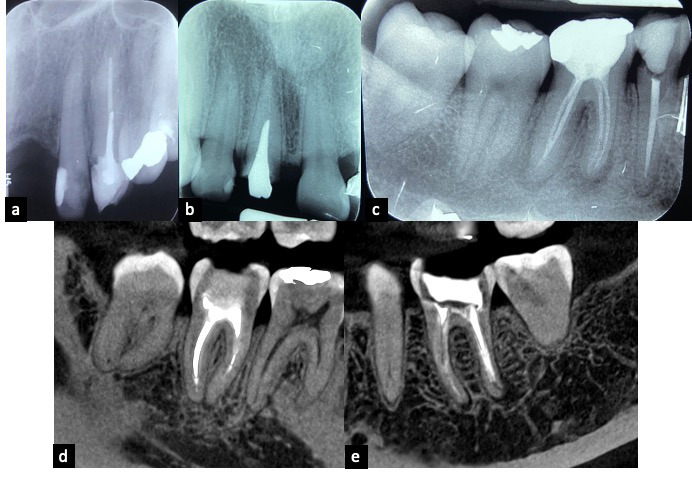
a) Radiografía periapical, diente 23; b) Radiografía periapical, diente 11; c) Radiografía periapical, diente 45 y 46; d) Imagen de CT, corte sagital de diente 47; e) Imagen de CT, corte sagital de diente 36. Dientes con conductos obturados asociados con algún tipo de cambio ósea estructural o rarefacciones que pueden estar relacionadas con procesos de curación prolongados.
